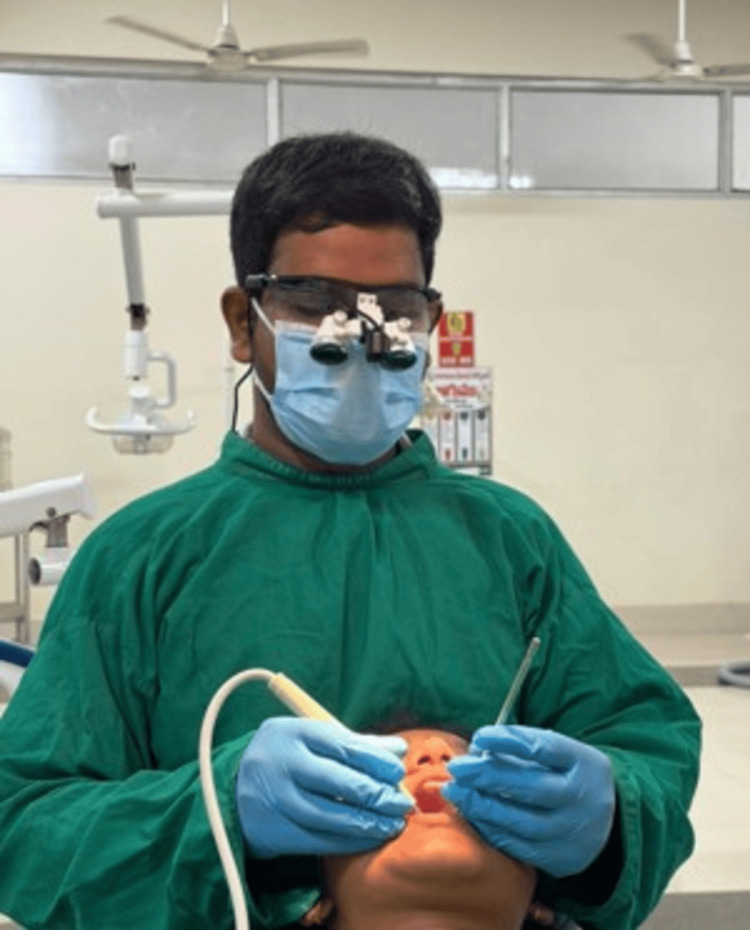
Ultrasonic scaling performed under magnification.
